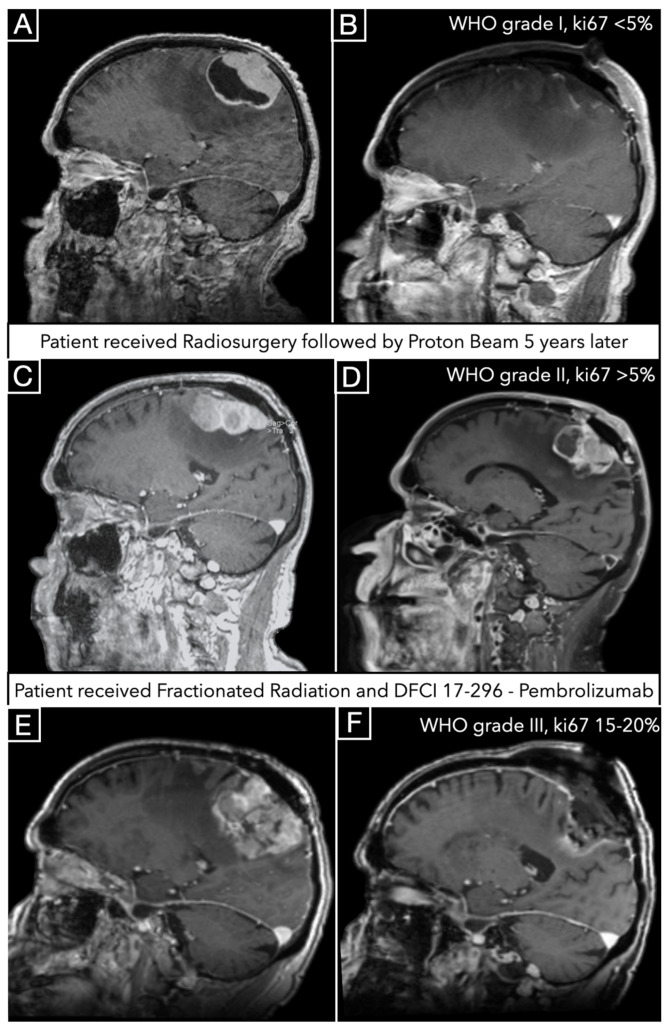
Preoperative and postoperative contrast-enhanced T1-weighted sagittal MR images following the first (A,B), second (C,D), and third (E,F) surgeries.
